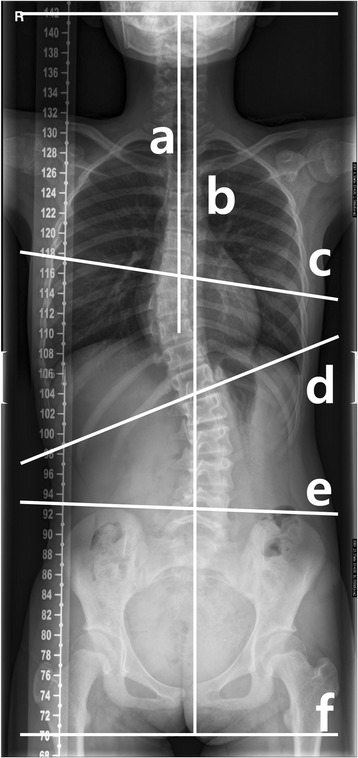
Radiographic measurements. The scoliosis Cobb angle was measured between c and d. Coronal balance was defined as the length between a and b. The angle of pelvic obliquity was measured between e and f. Apical vertebral translation was defined as the horizontal length between the center of apical vertebra and the C7 plump line. C7 plump line (a), center sacral vertical line (b), upper end plate of proximal end vertebra (c), lower end plate of distal end vertebra (d), line between both iliac crests (e), horizontal line (f)
